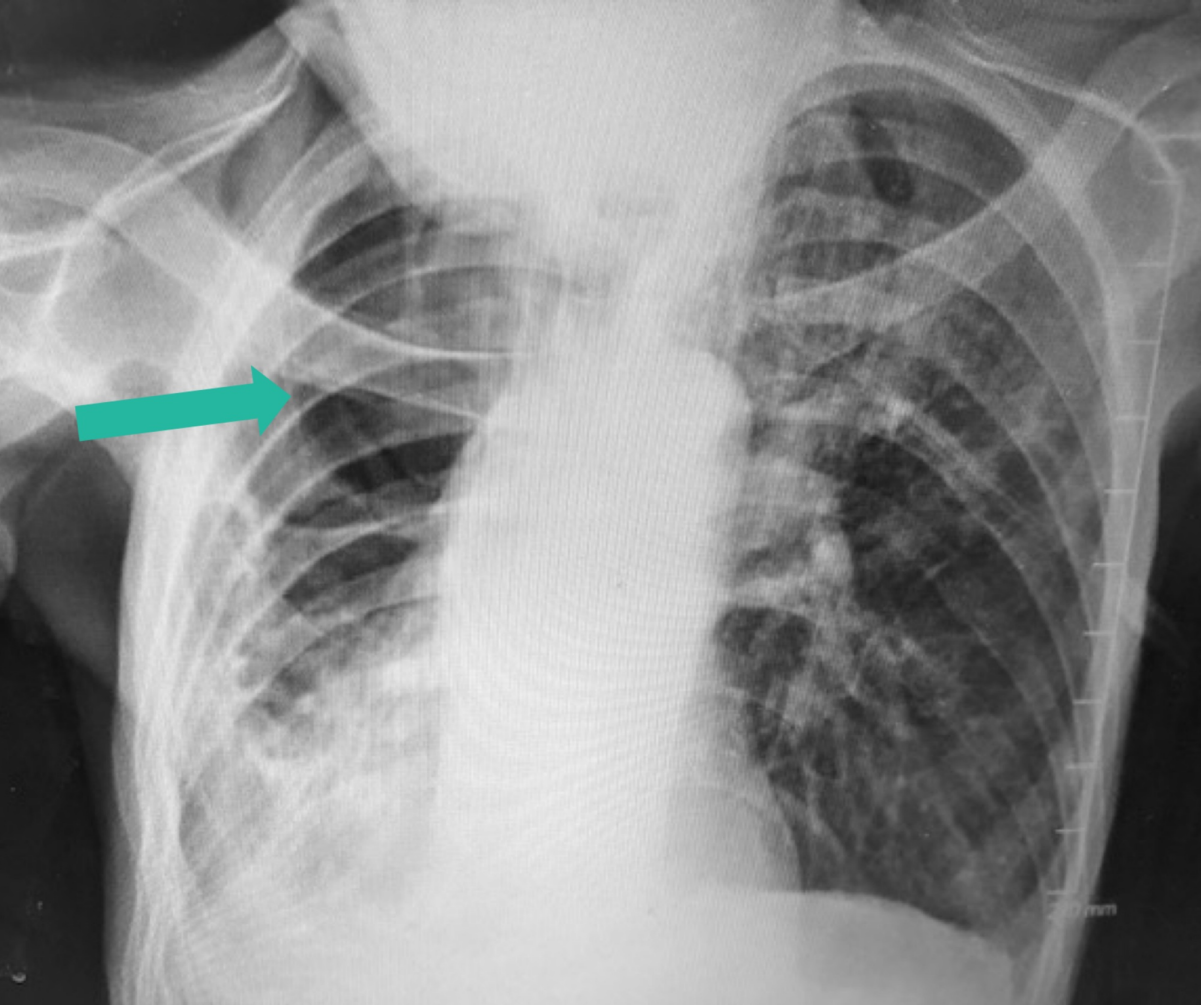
Chext X-ray on admission The patient’s chest X-ray showed left upper lobe and right basilar infiltration, with a cavitary process (green arrow) in right upper lobe.
